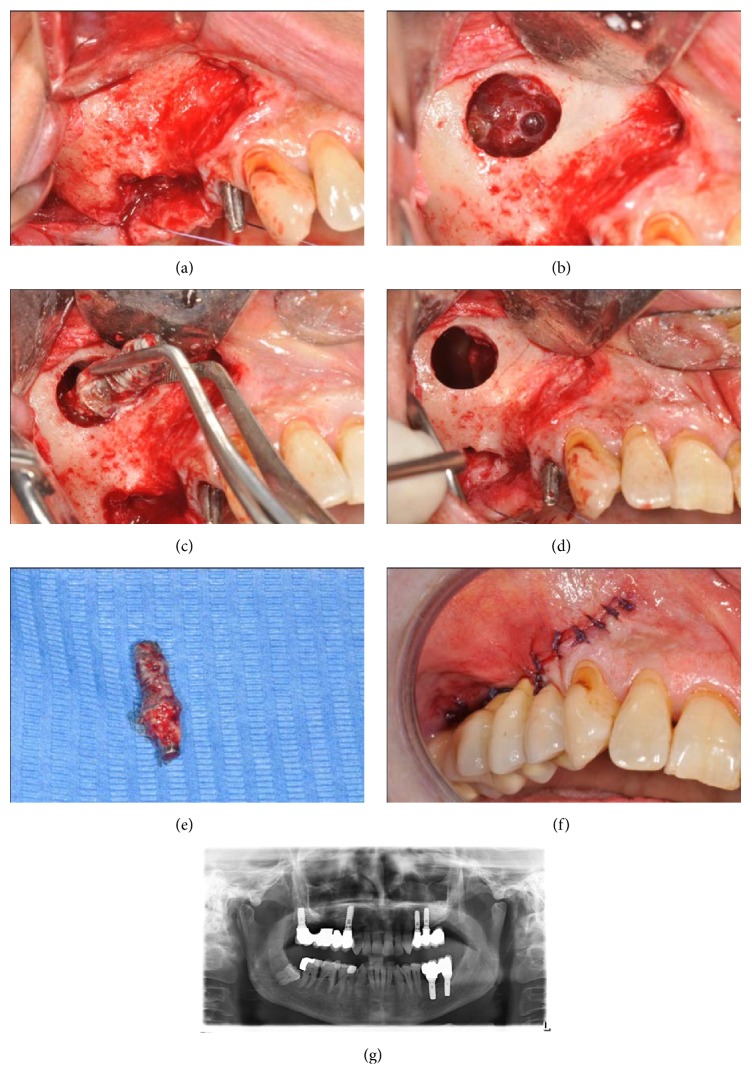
(a) Clinical situation after the flap was raised. (b) Photograph of the right maxillary sinus after a lateral window access showing sinusitis secondary to implant displacement. (c) Picture of the implant removal with forceps. (d) Maxillary sinus situation after its surgical revision. (e) Extra-oral view of the removed implant. (f) Clinical situation after the flap was sutured and the fixed prosthesis was cemented. (g) Radiographic view of the right maxillary sinus soon after the implant was removed.
